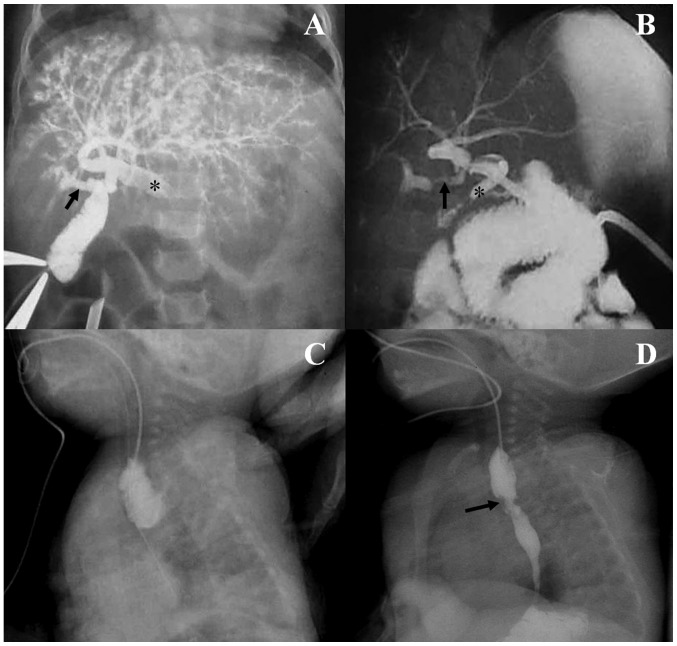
Cholangiography via punctured gallbladder and esophageal contrast radiography. Note the filling defect in the common bile duct (asterisk) and the dilated upstream biliary tract without contrast flow into the duodenum, and the accessory right hepatic duct (arrow) joining the cystic duct (A). A postoperative contrast study (B) via the T-tube placed into the common bile duct (asterisk) displayed a right bile duct stricture (arrow) where the gallbladder had been surgically removed. Note the free contrast passage into the duodenum. Esophageal contrast radiography (C) before gastroesophagostomy showed obvious dilatation of the upper esophagus, without contrast flow into the trachea, lower esophagus or stomach, while postoperative contrast radiography (D) displayed the contrast flow through a mild annular stricture (arrow) between the upper and middle esophagus.
